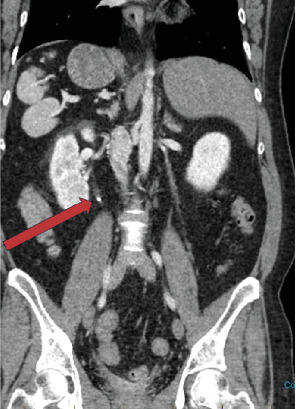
Coronal noncontrast CT demonstrates kidney stone in the right ureter (red arrow).
